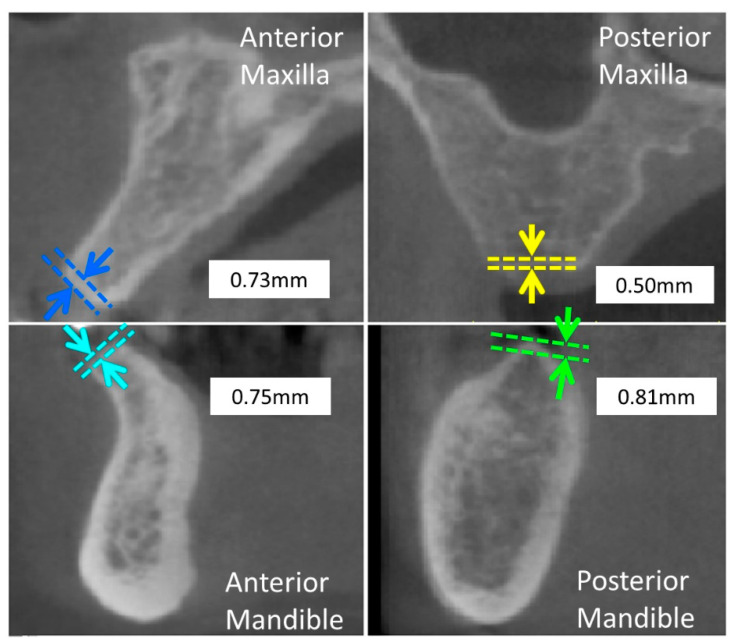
Thickness of crestal cortical bone at the dental implant site for the four jawbone regions, measured using cone-beam computed tomography (CBCT) images.
